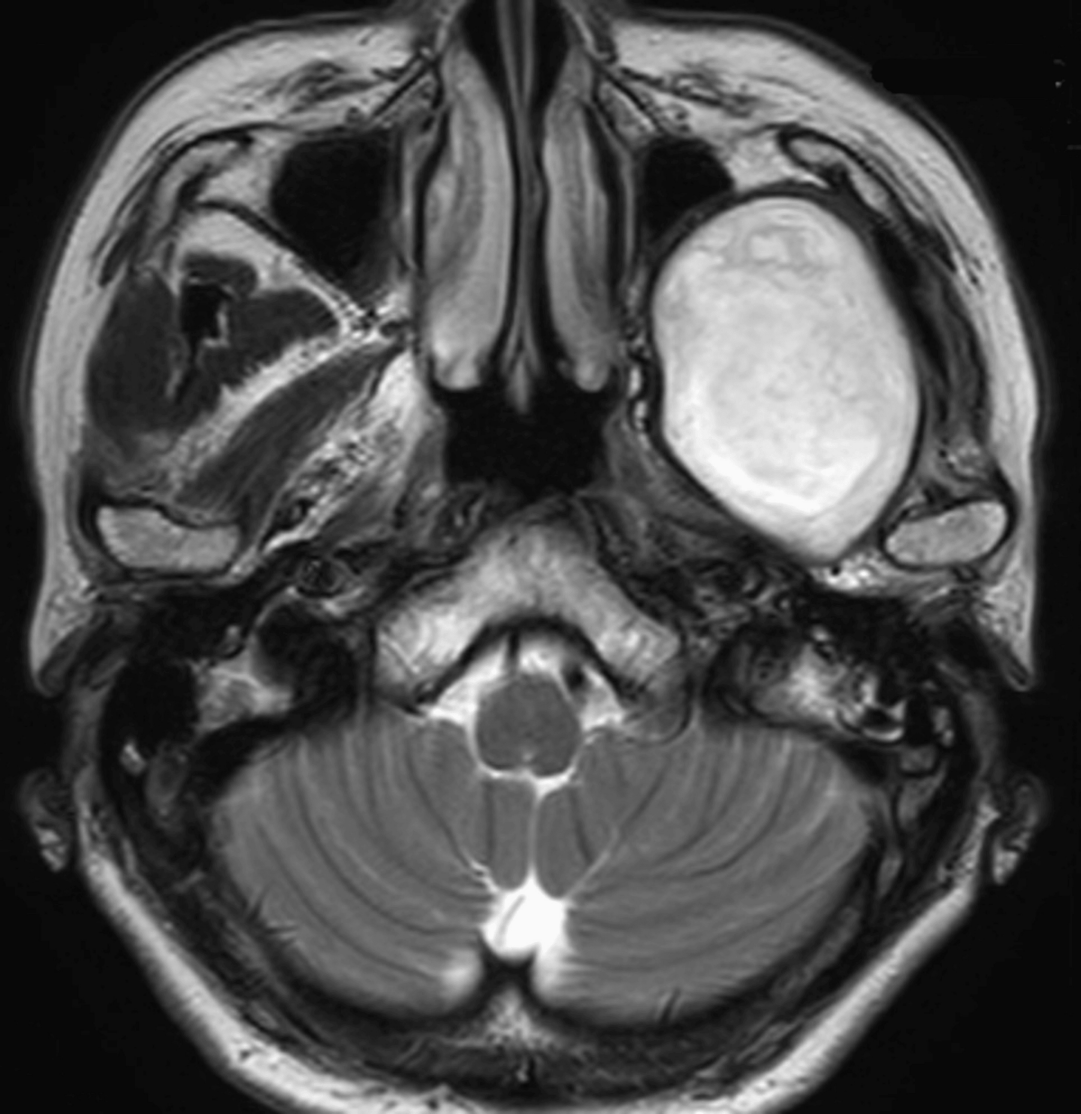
T2-weighed MRI in the axial view of Case 2.
